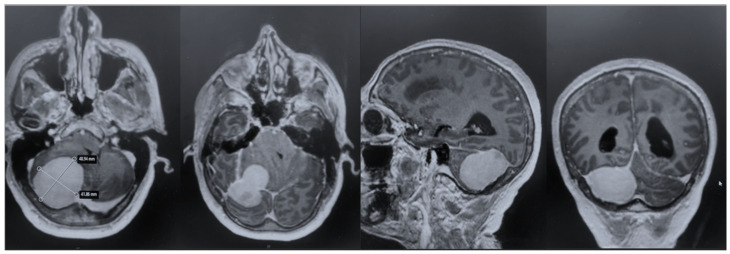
Preoperative MRI + C: a posterior fossa extra-axial mass was demonstrated, showing a low SI on T1 and intermediate SI on T2, which avidly enhanced post-Gad administration. The mass was broad-based on the right tentorium cerebelli with a discrete supratentorial extension, thus compressing the adjacent right cerebellar hemisphere, the brainstem, the IV ventricle, and the right occipital lobe.
